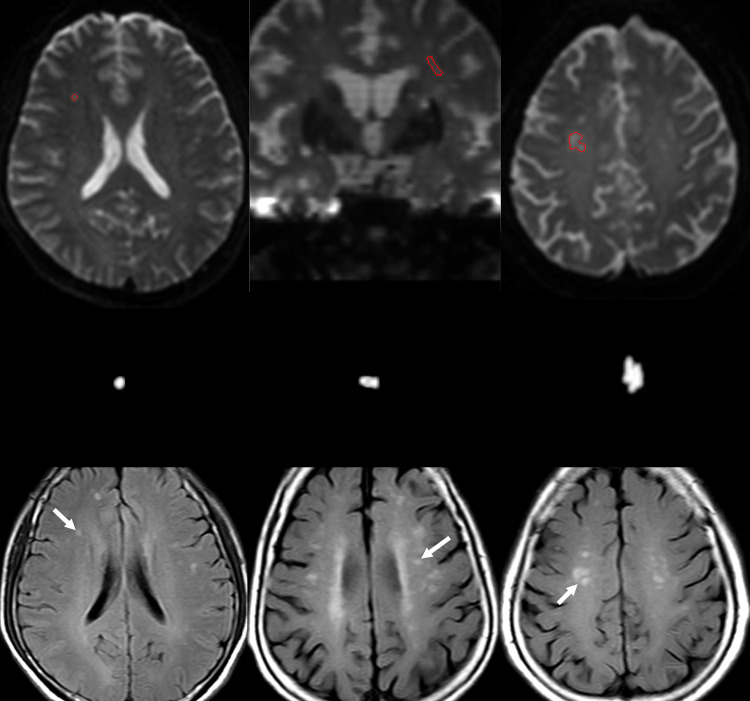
ROI determination of punctate, strip-shaped, and early fused lesions and the three-dimensional reconstruction after lesion segmentation. FLAIR images (bottom row) were used to differentiate WMH from perivascular spaces or lacunes.
